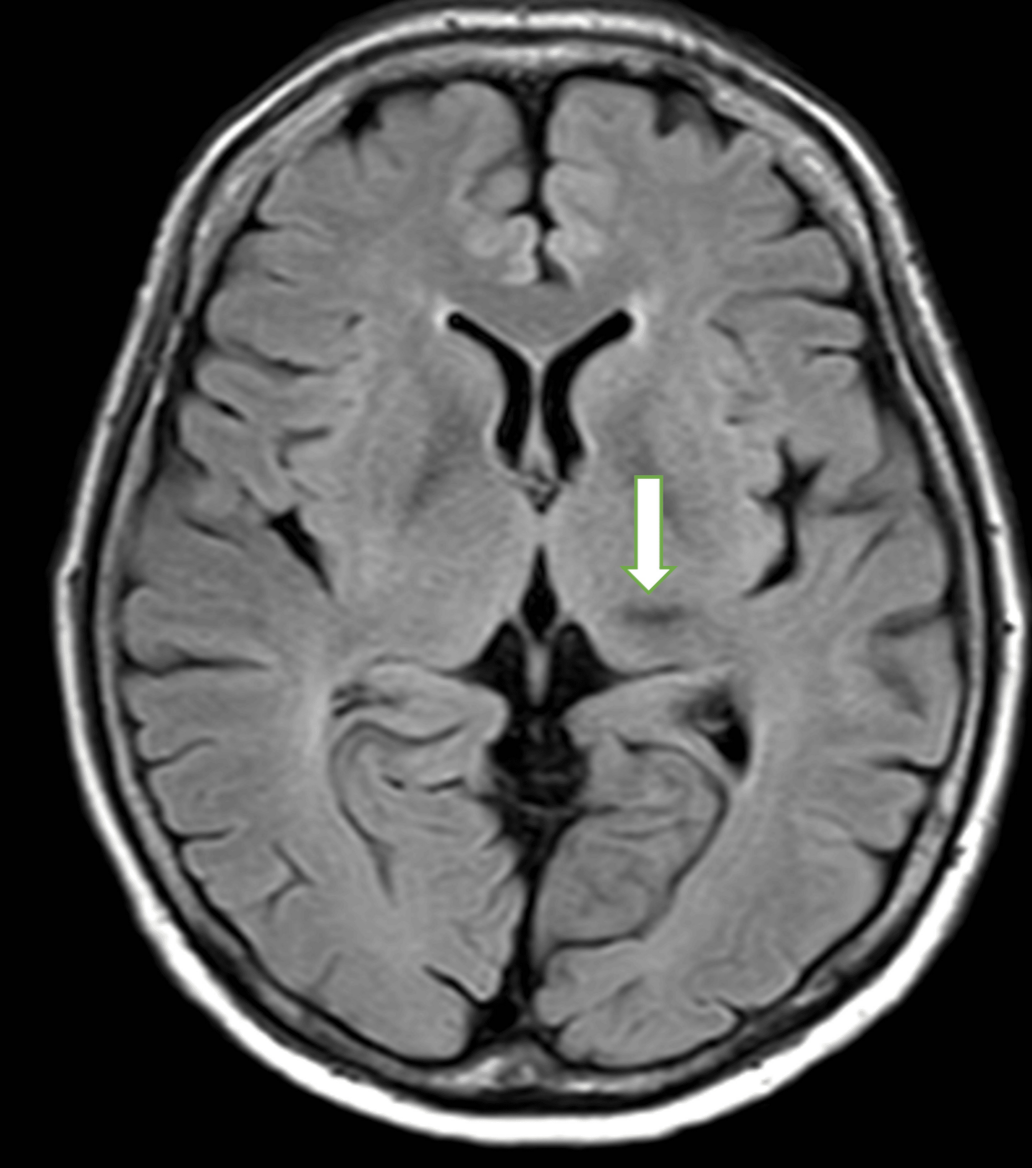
Head magnetic resonance imaging before elranatamab therapy. The arrow indicates hemosiderin deposition in the left thalamus, representing chronic changes from the previous cerebral hemorrhage.
